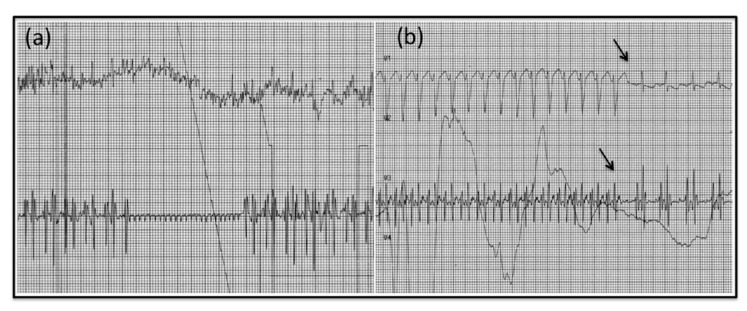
Transesophageal electrophysiological study. Lack of response to overdrive pacing (a) and absence of a blocked retrograde atriogram following the last beat of tachycardia (b) suggested automatic atrial tachycardia.
